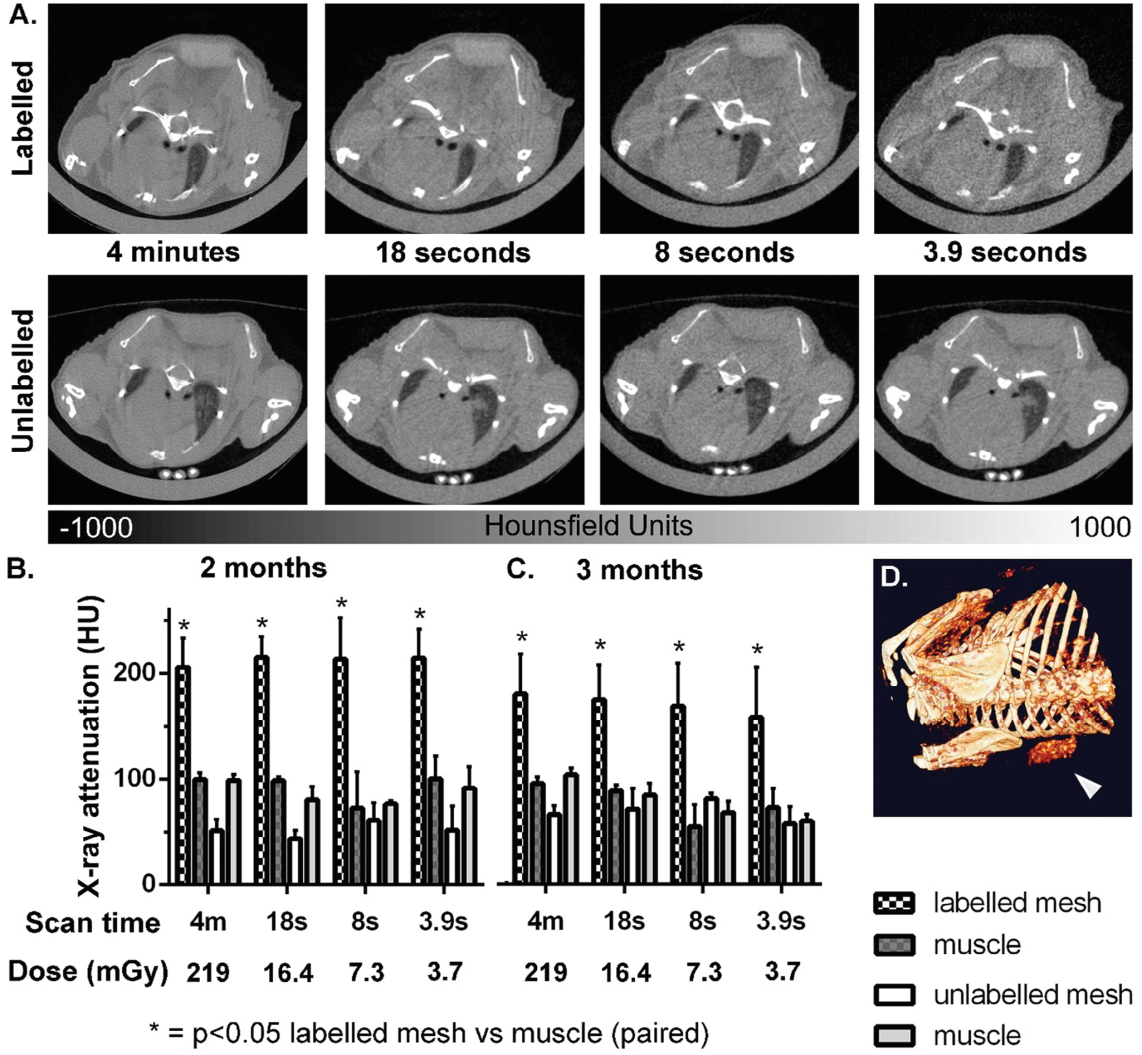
Labeled meshes retained their increased visibility versus muscle and control mesh with decreasing scan times, as shown here with A) axial CT sections at 3 months post‐implantation, and region of interest quantification of radiopacity at B) 2 months, and C) 3 months post‐implantation, with Holm‐Šídák multiple comparison tests. *N* ≥ 3 per time point, error bars show SD. D) Laterally migrated mesh visualized on a 3D rendered 18 s CT scan at 3 months post‐implantation using a clinically relevant X‐ray dose of 16.4 mGy.
